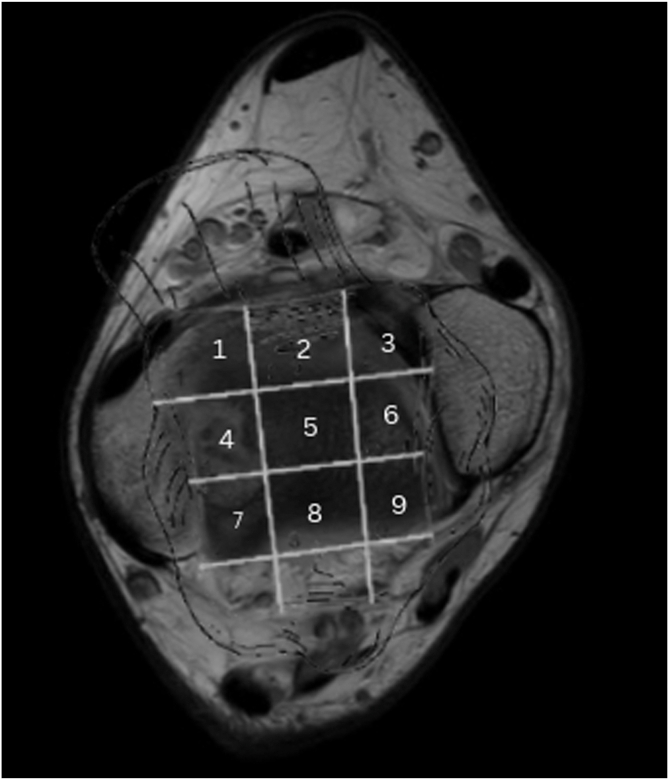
Raikin grid imposed on axial magnetic resonance imaging scan of talus. Lesions in locations 1, 4, and 7 are medial lesions; those in locations 3, 6, and 9 are medial lesions; and those in locations 2, 5, and 8 are central lesions.
